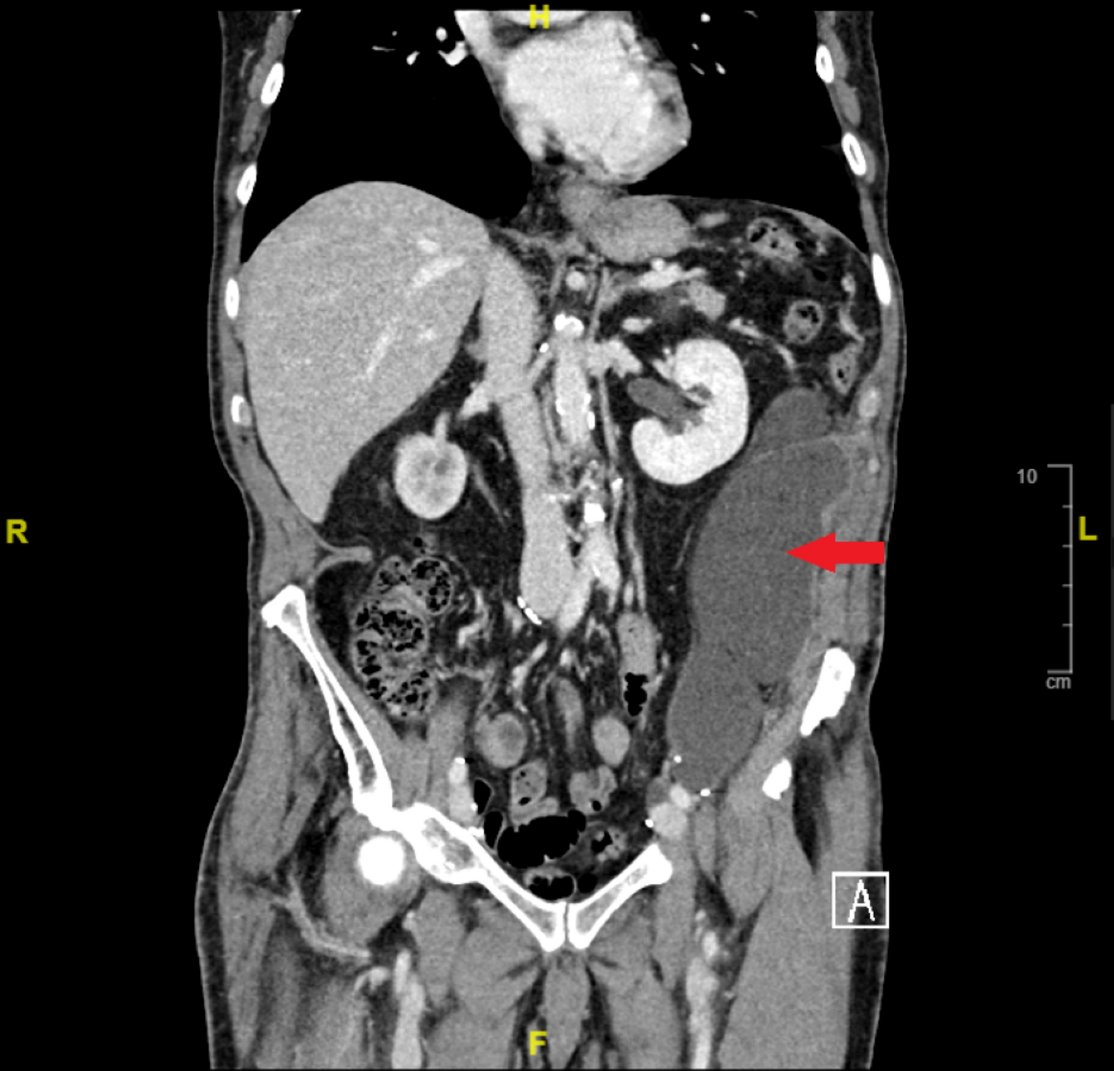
Computed tomography of the abdomen and pelvis Red arrow: Large left flank lymphocele measuring 20.4 x 9.8 x 6.2 cm with no associated gas formation or substantial surrounding inflammation.
